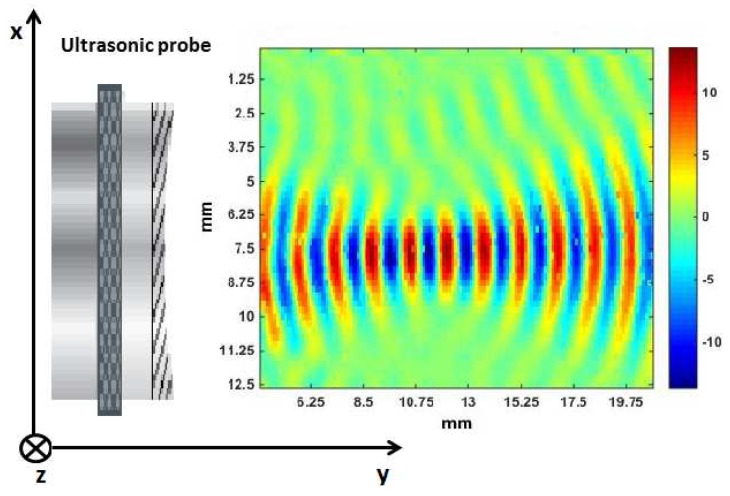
Laser Doppler vibrometry (LDV) visualization of the focused transducer Panametrics V303 for the frequency of 1 MHz. The ultrasonic probe is located on the left of the first measured wavefront.
